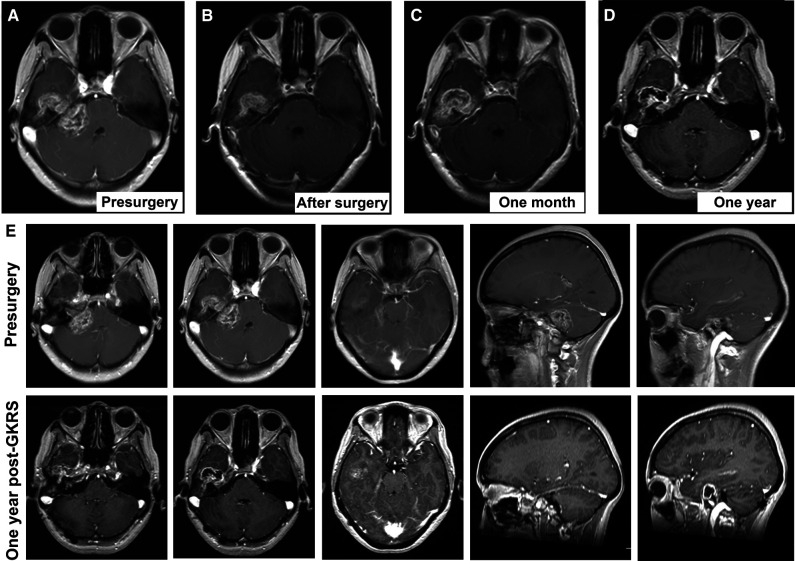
Consecutive follow-up MRI. (A) Presurgical scan; (B) MRI scan after microsurgery; (C) MRI scan one month after gamma knife radiosurgery; (D) MRI scan one year after gamma knife radiosurgery. (E) the comparison of MRI findings between pre-surgical scan and the scan one year after gamma knife radiosurgery.
